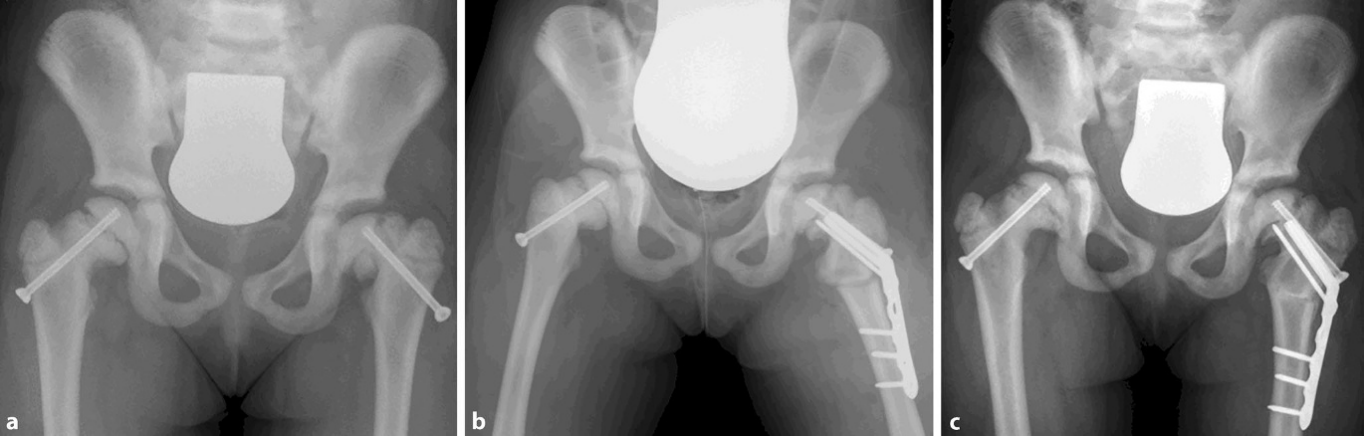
a Anteroposterior radiograph 7 months postoperatively showing a loss of fracture reposition on the left side with a coxa vara deformity and minor increased slippage of the epiphyseal plate. b Postoperative radiograph following revision surgery with re-osteosynthesis and a valgus osteotomy on the left side. c Radiograph 1 year postoperatively showing complete bony union of the osteotomy and no loss of correction at the femoral neck
